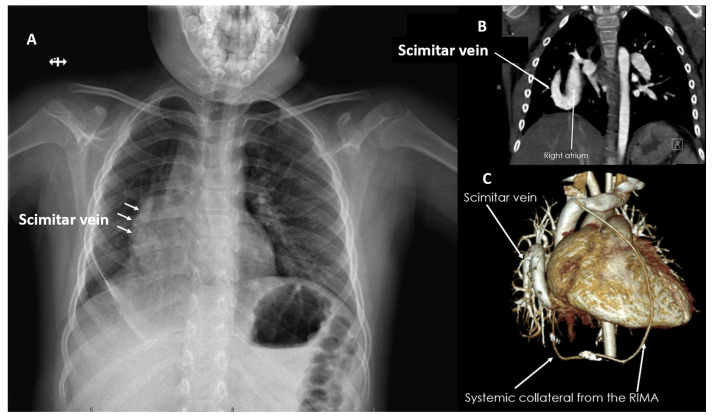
(A) A 6-year-old with scimitar syndrome. (B) Preoperative chest X-ray showing the scimitar vein, which was confirmed by computed tomography scan (C) and the 3D reconstruction showing the abnormal systemic collaterals to the right lung. RIMA: right internal mammary artery.
